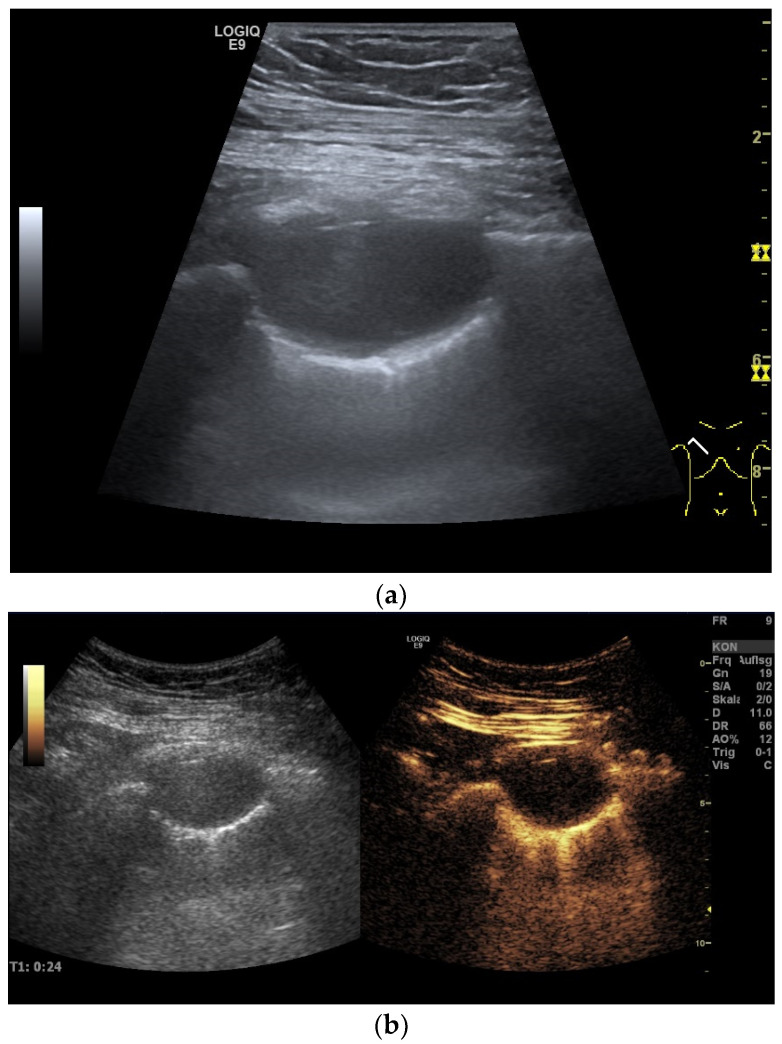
Encapsulated pleural effusion. A few months previously, a mitral valve replacement had been performed, which was accompanied by severe complications. The complications included a total right pleural effusion. No pulmonary mass was described preoperatively. In the case of pneumonia, a chest CT was performed, which showed a pleural mass in the right upper lobe. Initially, no distinction was made between a solid lesion and an encapsulated effusion, and image-guided sampling was recommended. A 38 × 24 mm hypoechoic to non-echoic well-circumscribed oval lesion in the right upper lung on high-resolution linear B-mode-ultrasound (a). The lesion did not show any enhancement in the CEUS at any time (b). A solid malignant tumor could, therefore, be excluded. In the US, the findings were, therefore, most likely attributed to an accumulation of fluid, an encapsulated effusion. The lesion was observed during the follow-up examination and regressed spontaneously over time, which was attributed to an encapsulated effusion.
